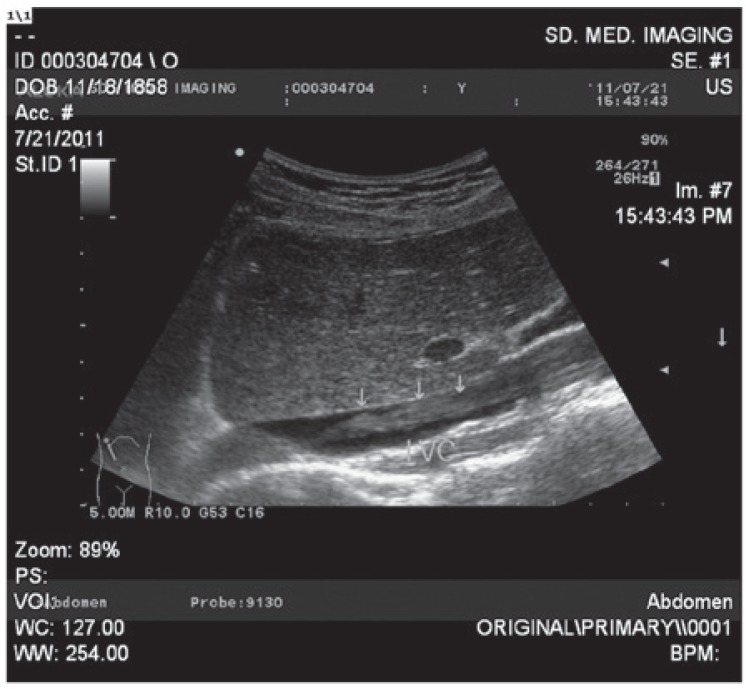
Abdominal US revealing a mobile solid mass in the inferior vena cava (IVC), which extended to the orifice of the right atrium. US, ultrasonography.
